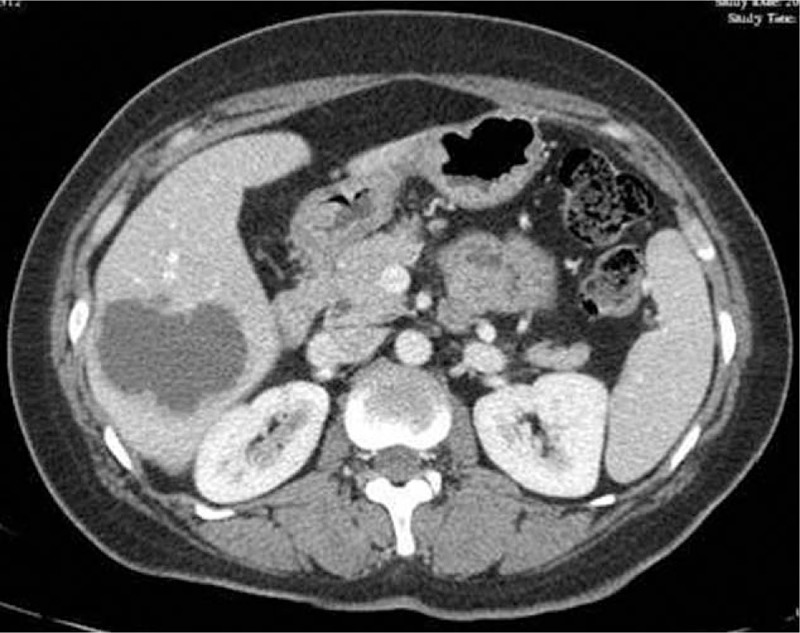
Computed tomography presenting a low density and heterogeneous lesion taking irregular wall and incompletely septa with strong contrast enhancement.
